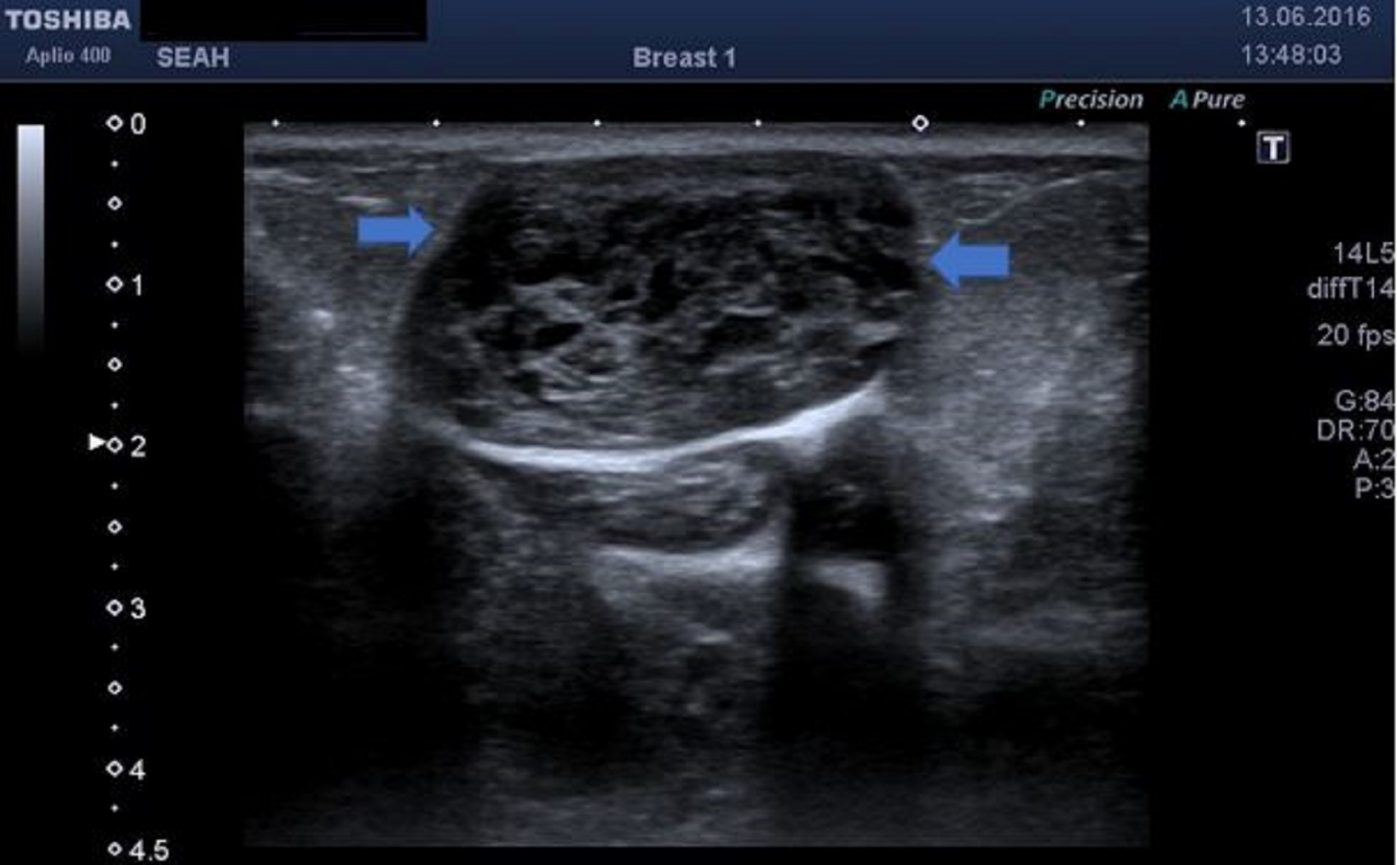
The ultrasonographic view of the cystic area
